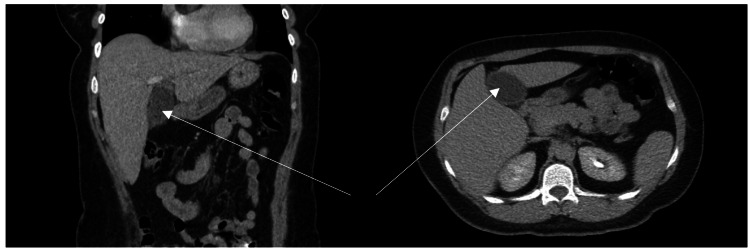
CT scan of the abdomen and pelvis Cholelithiasis with a mildly prominent gallbladder wall is observed. The arrows point to the gallbladder.
